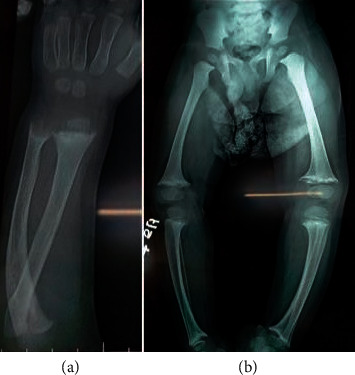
Radiographs showing cupping, fraying, and splaying of the distal radial, ulnar, femoral, and tibial metaphyses.
